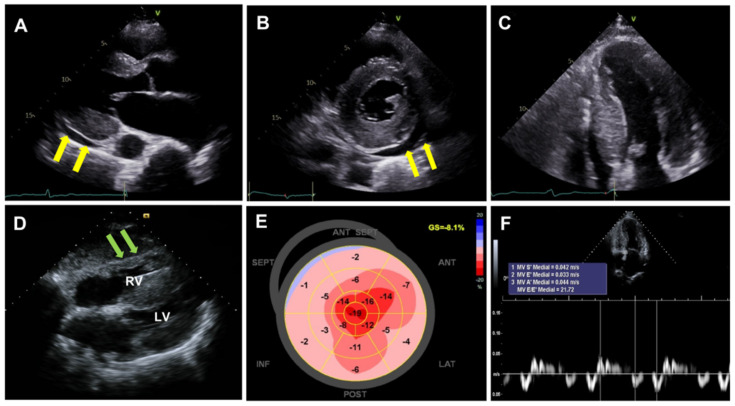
Echocardiographic findings of cardiac amyloidosis. (A–C): Thickened left ventricular wall (≥12 mm) with a granular sparkling appearance of myocardial texture and a small amount of pericardial effusion (yellow arrow), (D): right ventricular free wall hypertrophy (green arrow), (E): apical sparing of longitudinal strain (bull’s eye or cherry on top pattern), (F): deteriorated diastolic function.
